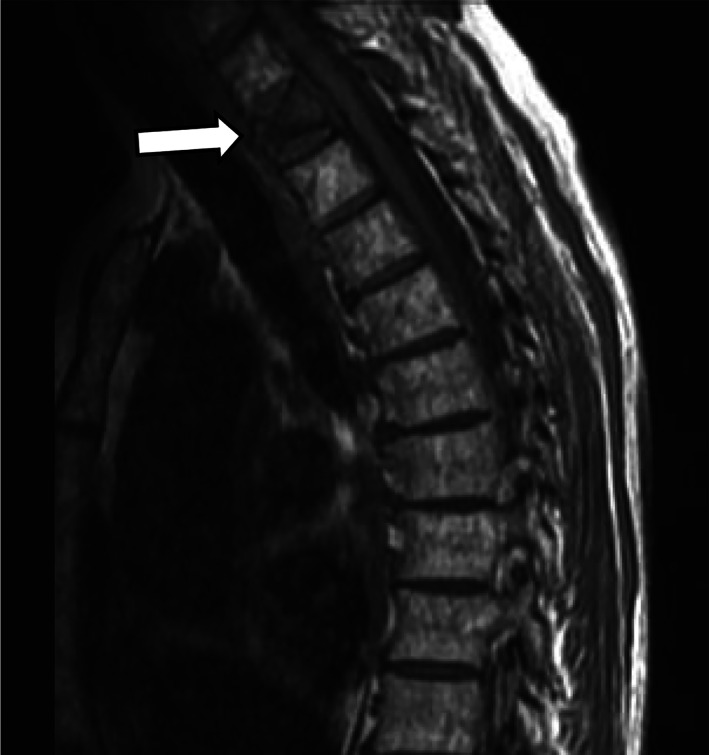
Magnetic resonance imaging of the spine showing pathologic fracture at T2 (arrow)
